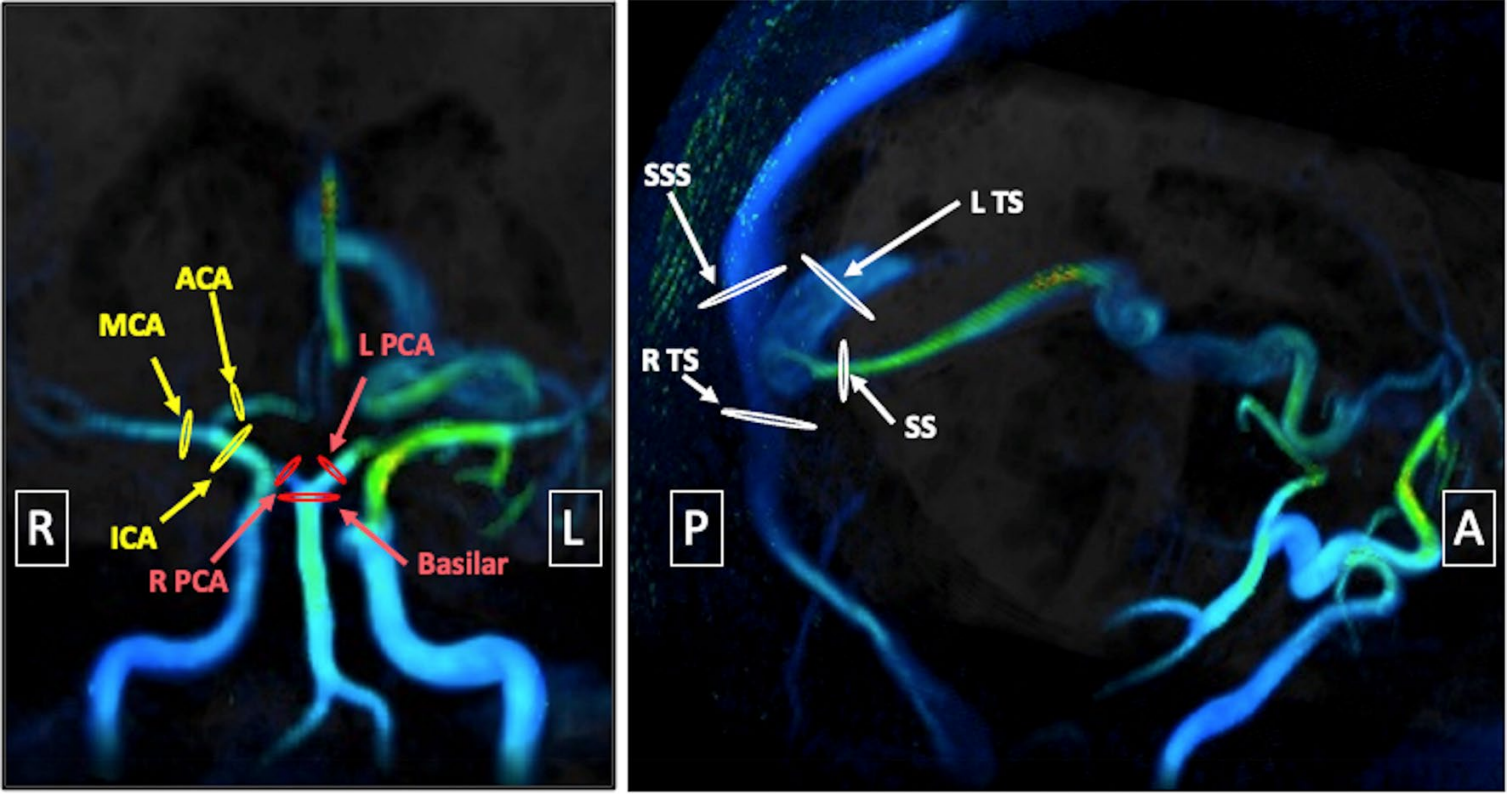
Coronal and oblique sagittal views of locations of blood flow measurement on 4D Flow MRI for a 19 year old male with a Spetzler Martin grade 2 AVM supplied by the left MCA. Locations of measurement in the anterior circulation are marked with yellow, posterior circulation are marked with red, and venous circulation are marked with white. AVM, arteriovenous malformation; ICA, internal carotid artery; MCA, middle cerebral artery; ACA, anterior cerebral artery; R PCA, right posterior cerebral artery; L PCA, left posterior cerebral artery; SS, straight sinus; SSS, superior sagittal sinus; R TS, right transverse sinus; L TS, left transverse sinus.
